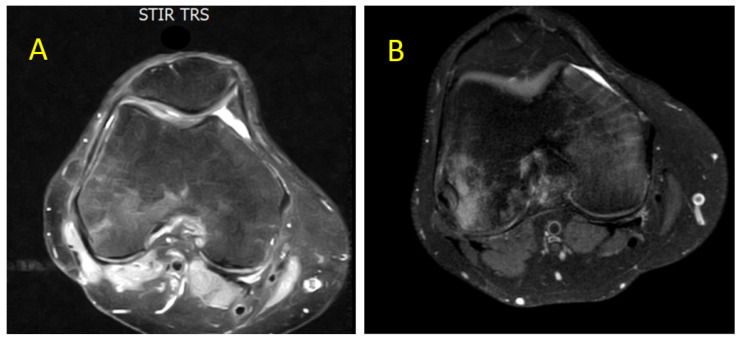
Axial MRI section of the knee showing gradual and progressive improvement of previously documented intra-spongious BME of the medial femoral condyle. (A) Partial resolution of BME after 2 months of laser therapy, TECAR therapy, bisphosphonates, and NSAIDs (April 2021). (B) Further improvement of BME after 4 months associated with clinical relief of bone-related symptoms (June 2021). Both images were acquired using STIR and T2-weighted fat-saturated sequences. ABBREVIATIONS: NSAIDs (nonsteroidal anti-inflammatory drugs) and TECAR (capacitive and resistive electric transfer).
